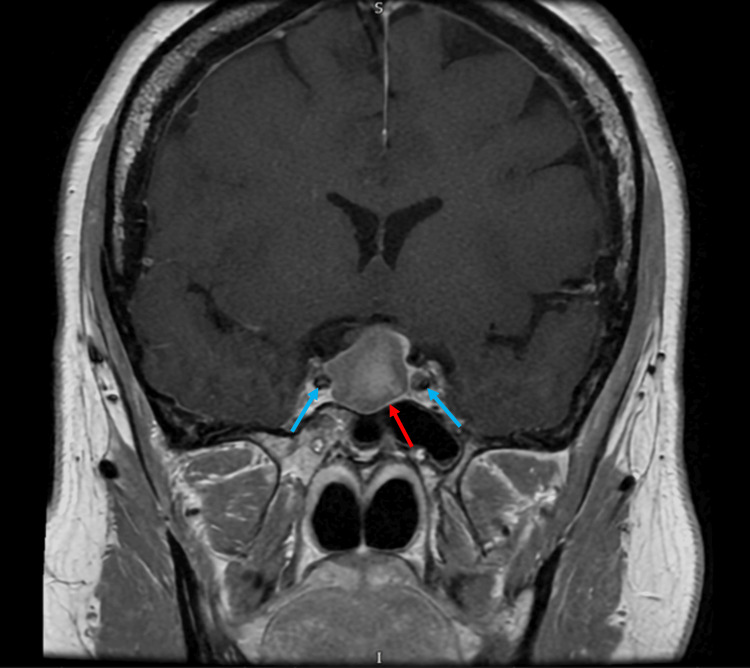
T1‑weighted, contrast‑enhanced coronal MRI demonstrated a 21 × 13 mm sellar mass (red arrow) with high T1 signal intensity, no measurable contrast enhancement, and suprasellar extension abutting both internal carotid arteries (blue arrows).
